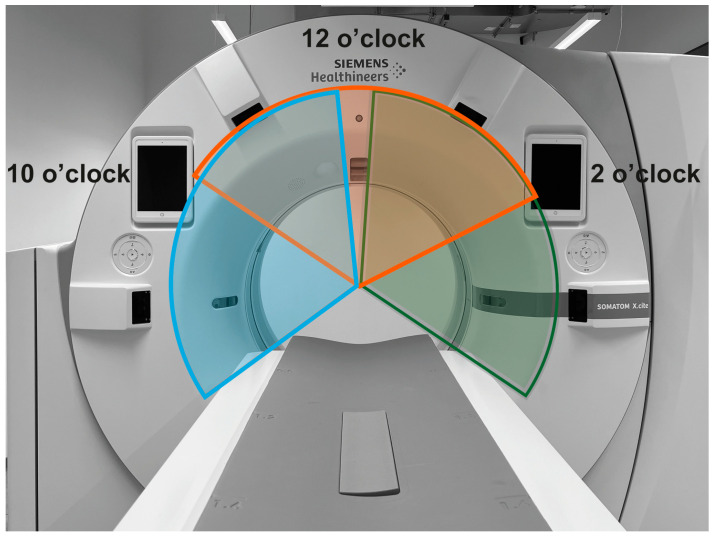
Angular segments with X-ray tube current switched off (blue: 10 o’clock segment, orange: 12 o’clock segment, and green: 2 o’clock segment).
